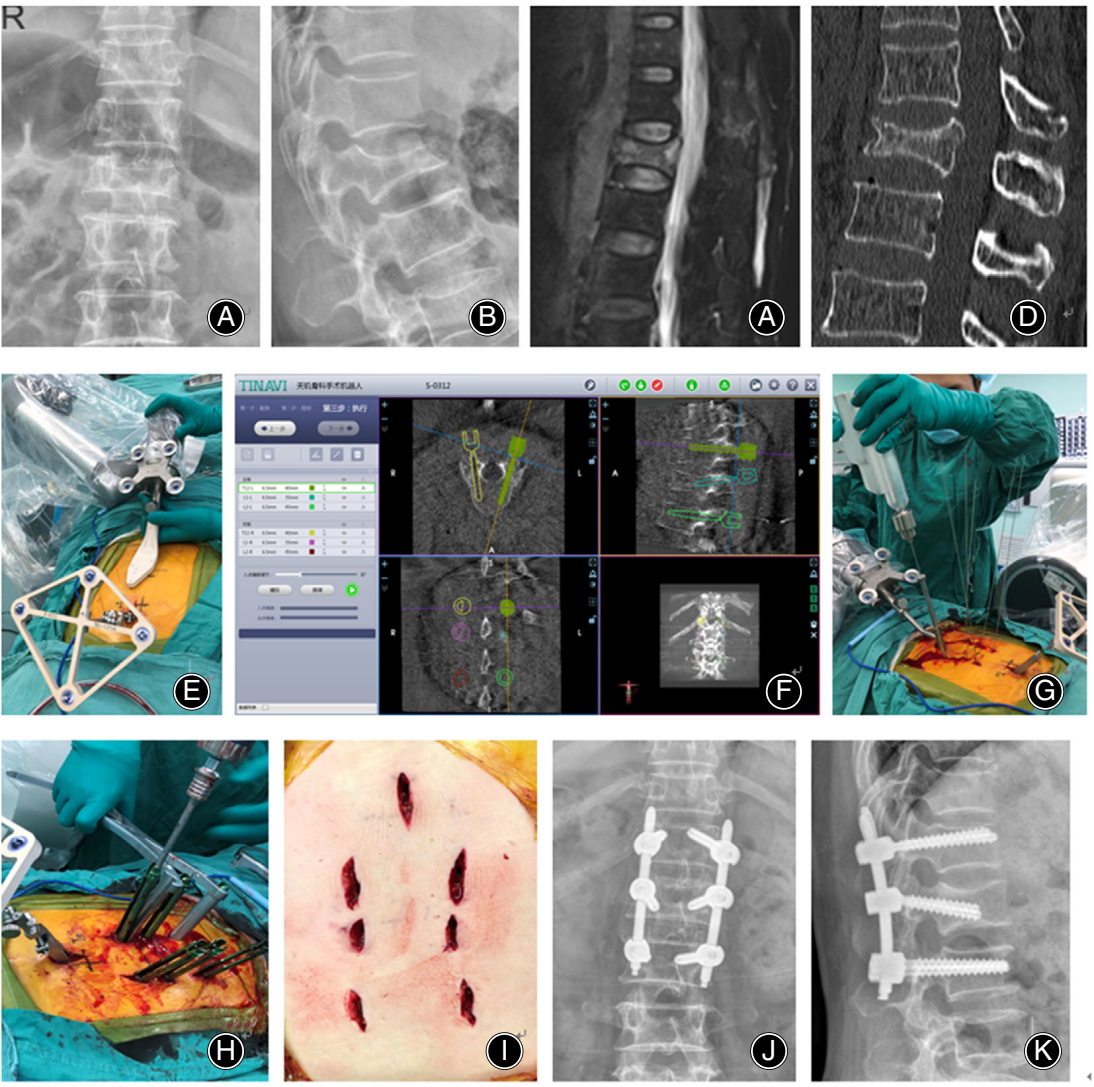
The TiRobot‐assisted procedure used for a 62‐year‐old woman with an L_1_ vertebral compression fracture and posterior ligamentous complex injury. (A) Preoperative anteroposterior radiograph. (B) Preoperative lateral radiograph. (C) Preoperative magnetic resonance image. (D) Preoperative computed tomography (CT) scan. (E) Robot registration. (F) Planning. (G) Insertion of the guide pin. (H) Reduction. (I) Postoperative incision. (J) Postoperative anteroposterior X‐ray image. (K) Postoperative lateral X‐ray image
